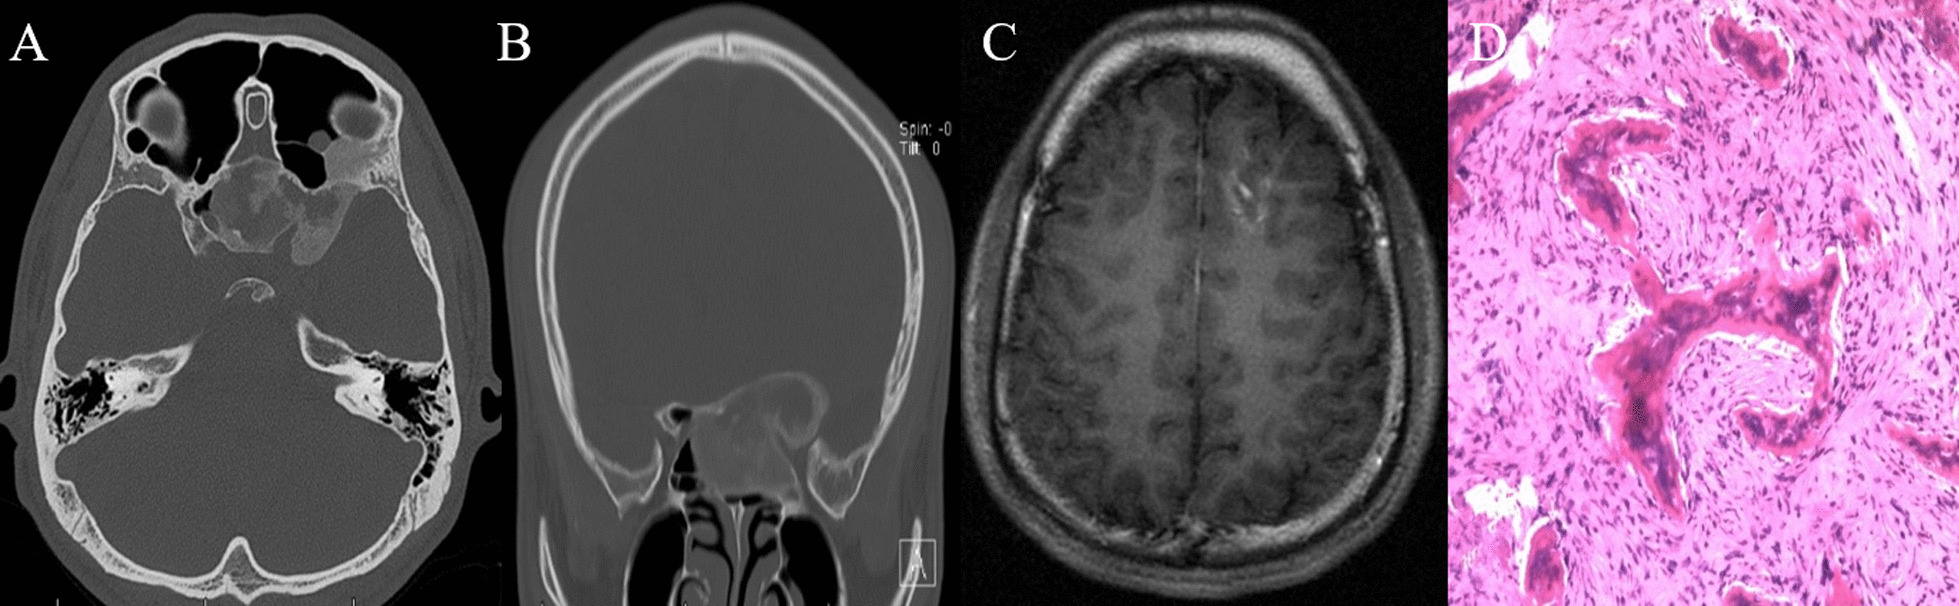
CT showing FD of sphenoid bone and left orbital apex (A, B). MRI showing venous malformation of the left frontal lobe (C). The patient’s FD was managed with transnasal surgery and the pathological report confirmed the FD diagnosis (D)
